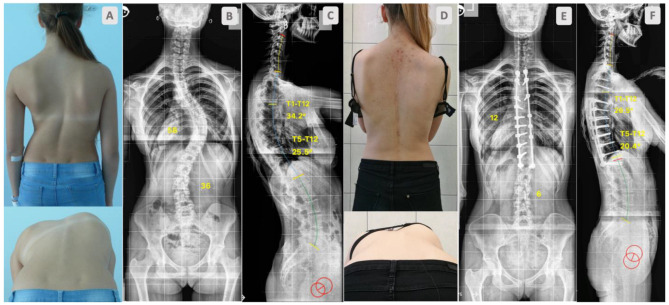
Fourteen-year-old girl with adolescent idiopathic scoliosis. Preoperative clinical pictures (A), standing radiographs, AP (B), lateral (C). Outcome at 2-year follow up after surgical scoliosis correction with DRC and DVR. Clinical pictures (D), standing radiographs, AP (E), lateral (F).
